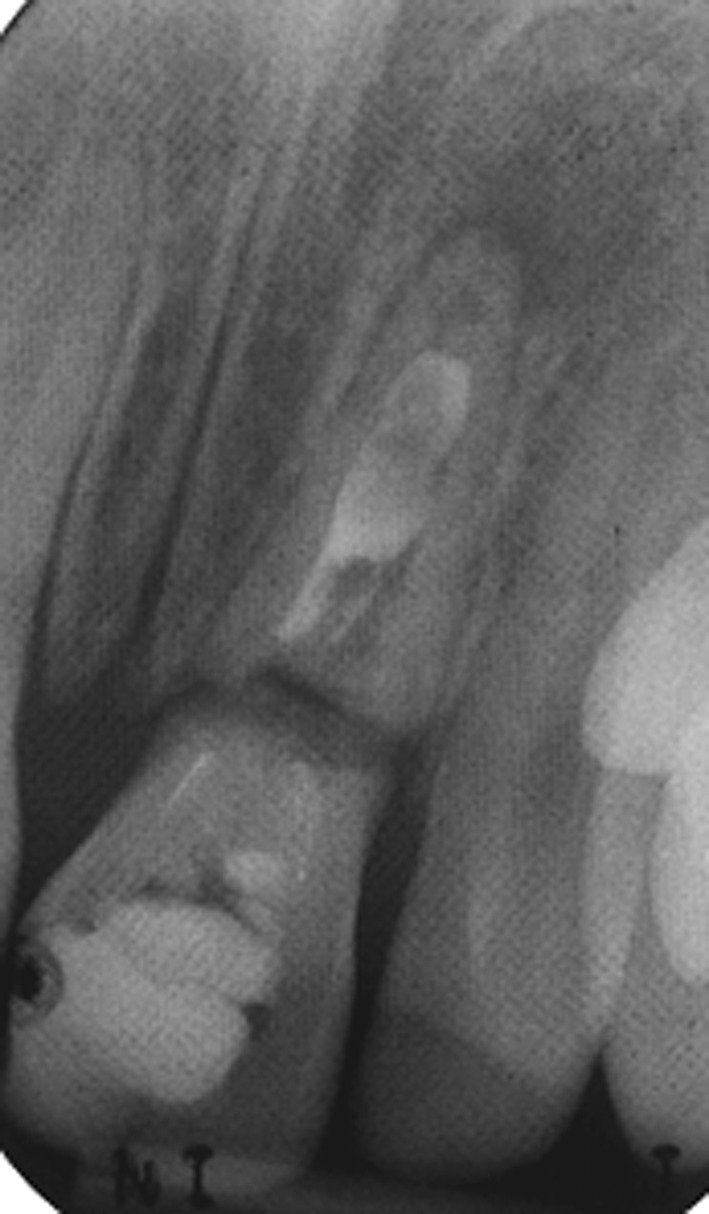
Periapical radiograph showing cervical root fracture of a tooth treated with calcium hydroxide apexification
